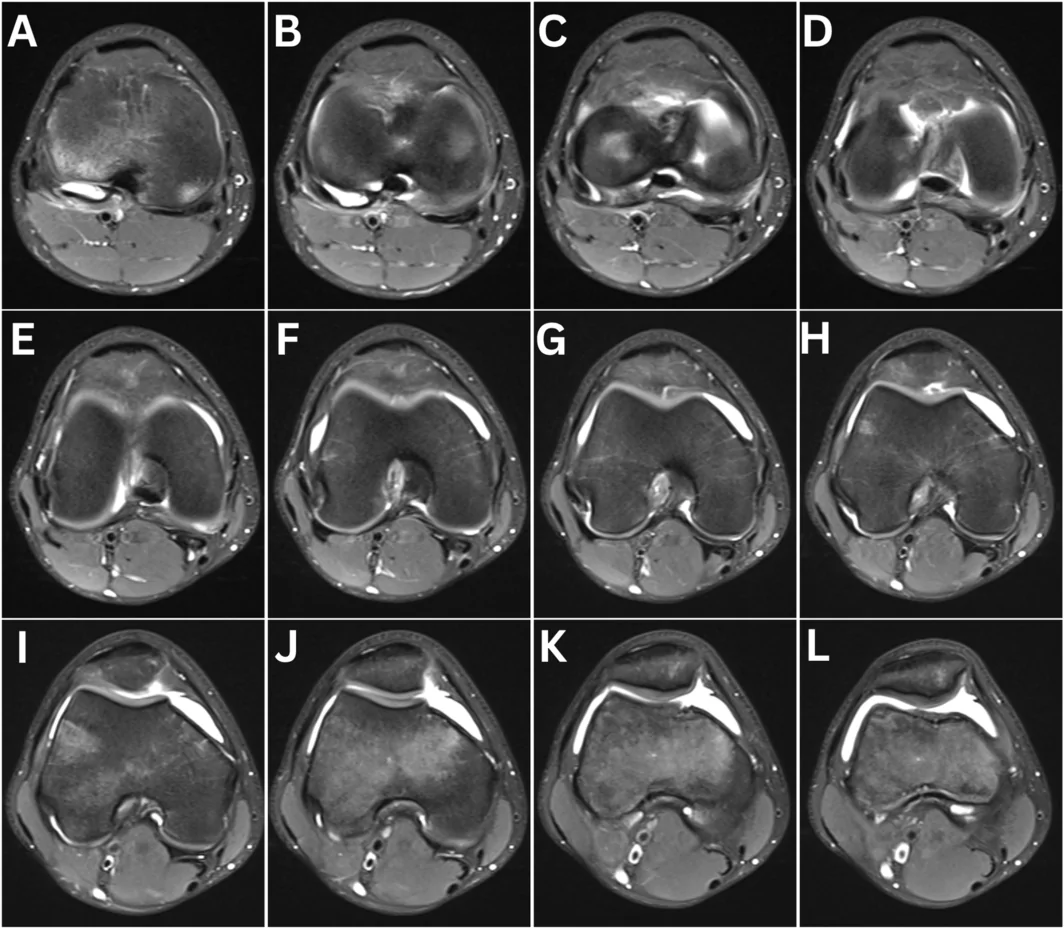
Initial axial MRI. Axial T2‐weighted fat‐suppressed images (A–L, proximal tibia to distal femur) show absence of the normal ACL band, with heterogeneous high signal in the intercondylar notch consistent with a torn, edematous ligament. Associated pivot‐shift bone bruising of the lateral femoral condyle and posterolateral tibial plateau, with joint effusion, is present.
